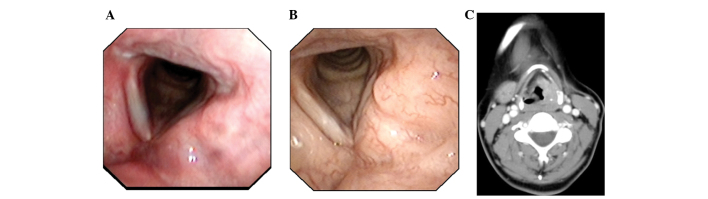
(A) Fiber optic laryngoscopy images identifying chronic congestion of the bilateral vocal folds and swelling of the left ventricular fold. The mucosa of the bilateral pyriform fossa was soft and no neoplasm was observed (18 months prior to the first surgery). (B) No obvious change one week prior to the first surgery. (C) Computed tomography of the larynx showing asymmetry of the bilateral posterior structure, an enlarged laryngeal wall beside the left pyriform fossa and a soft-tissue mass with obscure margins involving the left ventricular fold, the anterior commissure and extending to the pyriform fossa.
